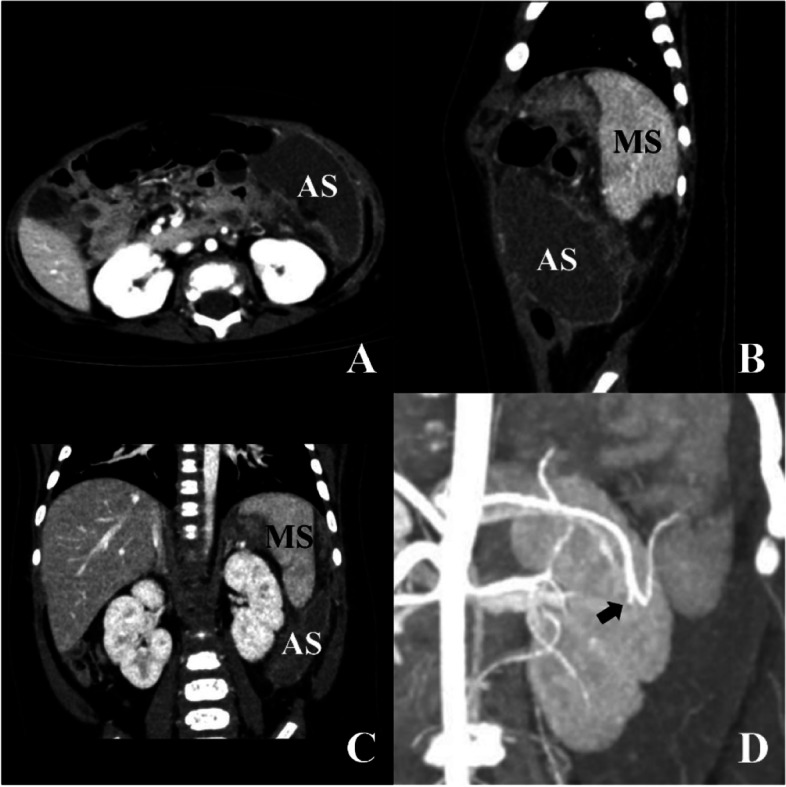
Preoperative contrast CT result of case 6, showing accessory splenic torsion with infarction (AS = accessory spleen, MS = main spleen). Rim sign (enhancement of the capsule with non-enhancement of the parenchyma) of the accessory spleen was obvious, indicating infarction in A (cross sectional view), B (sagittal view) and C (coronary view). The main spleen was at normal location with normal perfusion in B and C. The accessory splenic artery originated from the main splenic artery and was interrupted soon after branching in D (black arrow)
